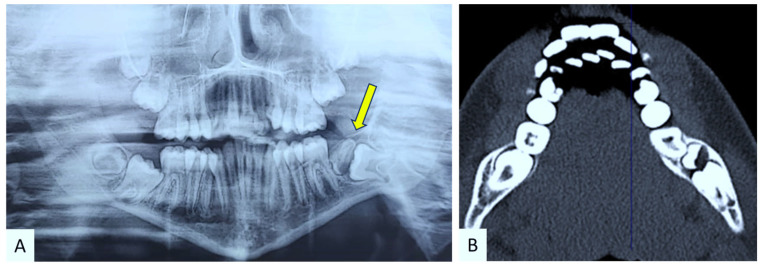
Panoramic radiograph showing a radiopaque/radiolucent lesion overlining a retained tooth (better highlighted in yellow) (A) consisting of the developing tooth crown with a radiopaque appearance encompassing the radiolucent lesion, better defined on CT scan (B).
